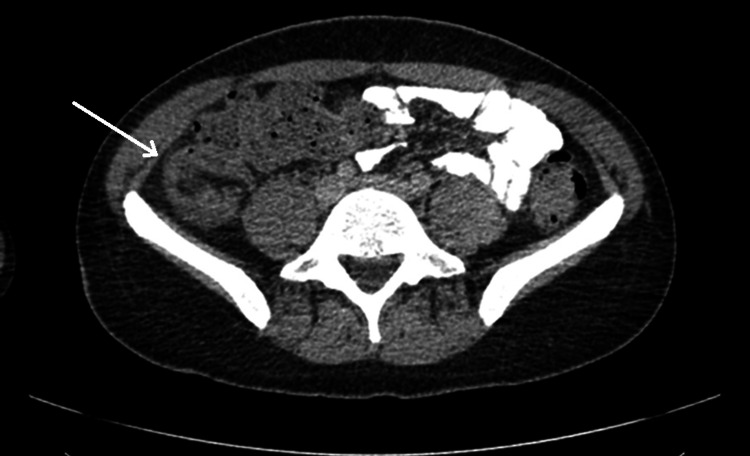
CT of chest, abdomen, and pelvis with contrast Axial image of CT chest, abdomen, and pelvis: the arrow points to a thickened, inflamed appendix in the right lower quadrant, measuring 12 mm in diameter, posterior to the cecum with periappendiceal fat stranding and small local lymph nodes, consistent with acute appendicitis. There is no evidence of collection, generalized peritonitis, free fluid, or free gas. Also, there were bilateral small-volume cervical lymph nodes that were nonspecific, bilateral tonsillar hypertrophy, but there was no evidence of lymphoma, lymphoproliferative disorder, or sarcoidosis (not seen in the figure) CT: computer tomography
